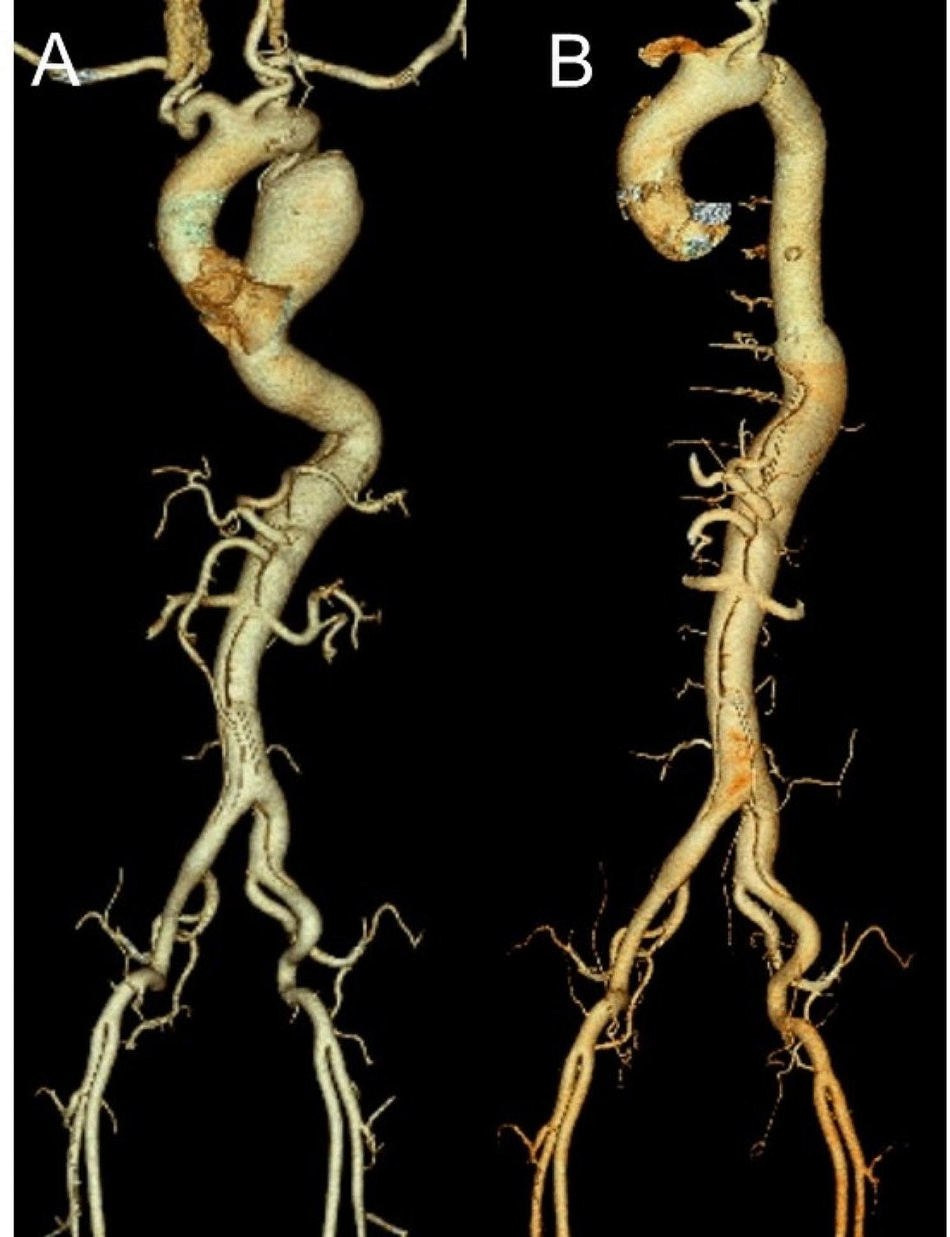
Preoperative computed tomography angiography reveals the dissecting aortic aneurysm in the descending aorta distal to the left subclavian artery (A) Postoperative computed tomography angiography shows replacement of the dissecting descending aorta with a vascular graft (B)
